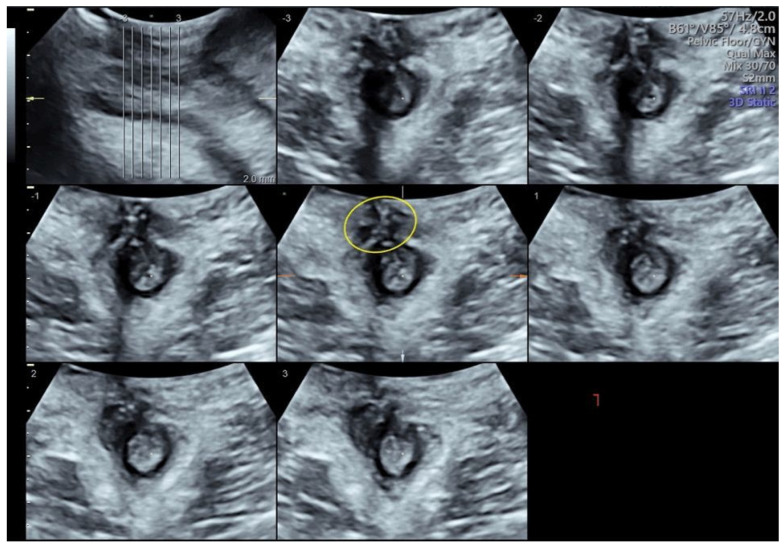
Three-dimensional transperineal ultrasound performed one month after secondary surgery. The anal sphincter repair is indicated by the yellow circle.
